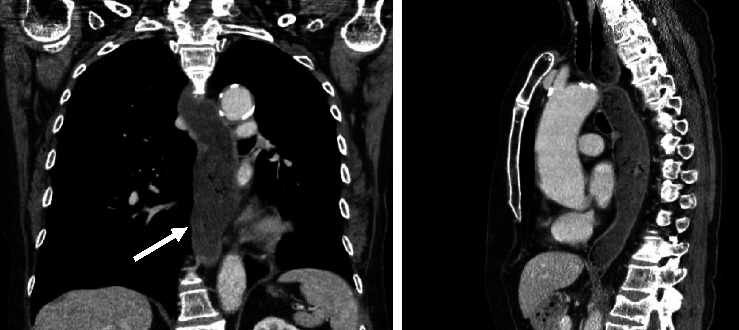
CT scan 2^nd^ of April 2020, Toshiba Aquilion 64, contrast agent Accupaque 350, and venous phase.
